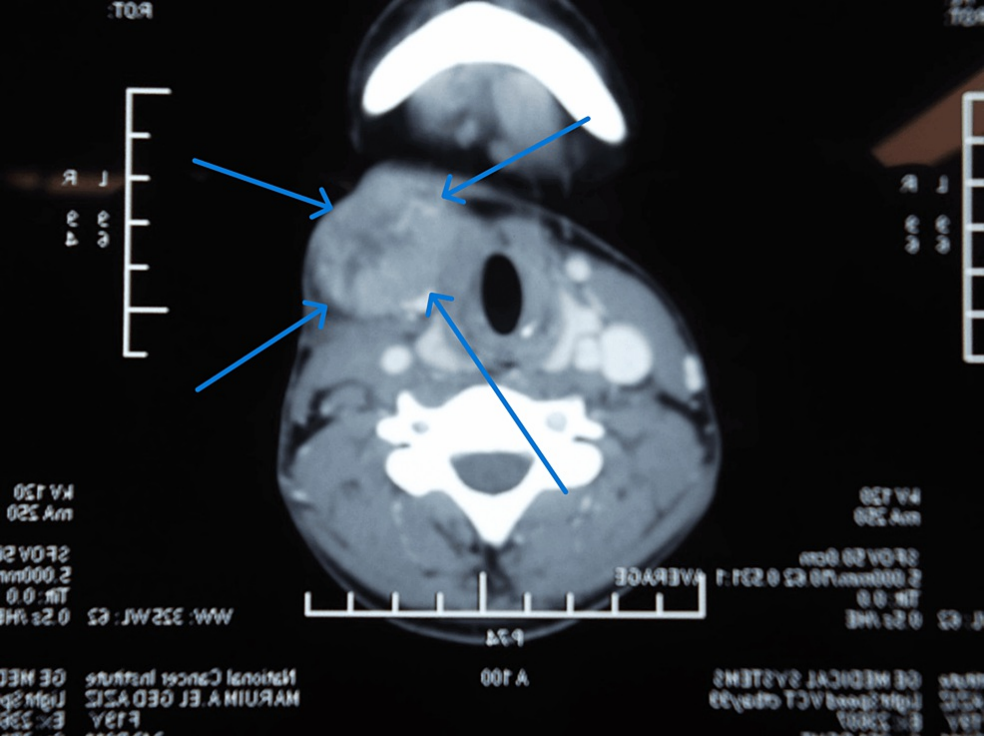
CT scan with contrast of one of our cases with recurrent carotid body tumor
